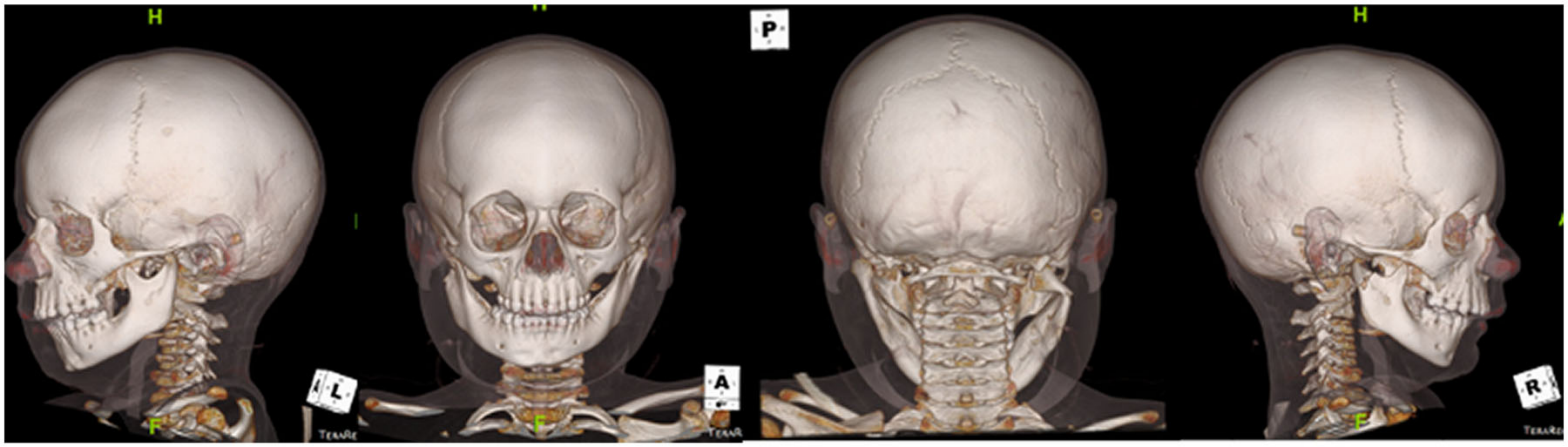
CT craniomaxillofacial findings revealed a complex, tripartite mandibular fracture, including bilateral condylar fractures with lateral dislocation of the left condyle and a symphyseal fracture. Specifically, there was a comminuted left condylar fracture with medial and inferior displacement of the fracture fragments as well as lateral and inferior dislocation of the intact lateral aspect of the condyle; near complete right subcondylar fracture with lateral apex angulation as well as medial and inferior dislocation of the right mandibular condyle; a mildly distracted oblique left parasymphyseal fracture; retrognathism; and, associated perimandibular soft tissue edema with mild subcutaneous edema on the right, and a 1.0 cm hematoma adjacent to the left mandibular condyle.
